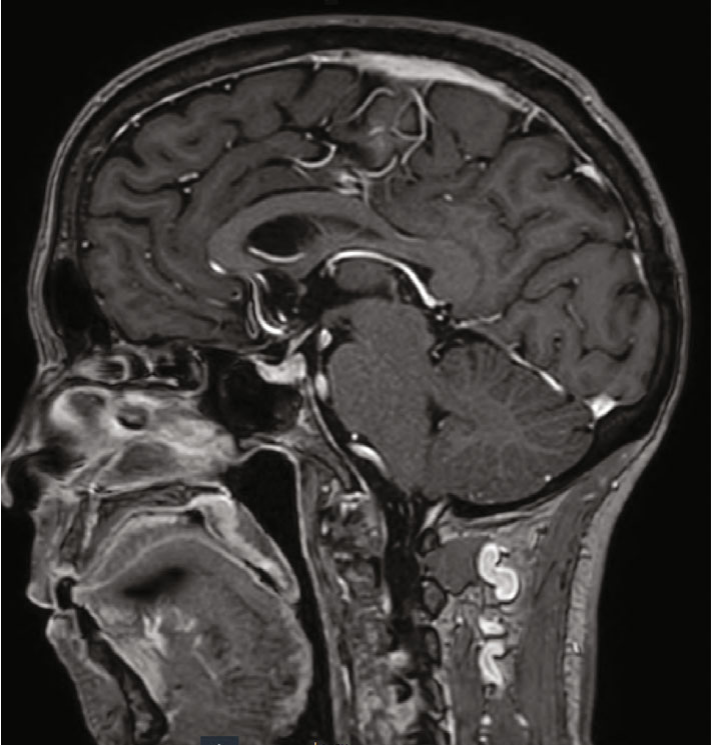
MRI done 4 weeks later shows no abnormalities.
